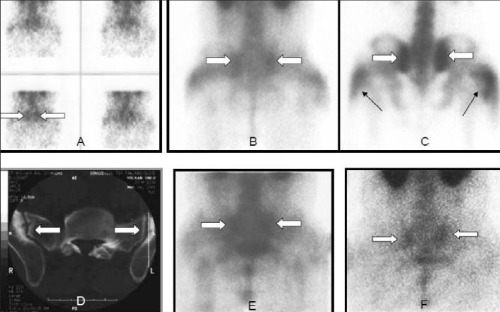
A 32-y-old male with clinically active sacroiliitis. Flow (A),blood pool (B), 4^th^ hour planar (C) images of bone scan. CT (D), 4thhour (E) and 24^th^ hour (F) HIG images of pelvis. Note the increasedflow, hyperemia and osteoblastic activity in sacroiliac joints on bonescintigraphy (white arrows). Increased HIG accumulation andretention were concordant with the clinical findings in this patient.Degenerative changes of both sacroiliac joints accompanied withsubchondral sclerosis and small calcifications were reported on CT(white arrows). The bilateral gluteal uptake is due to the repeatedintramuscular injections (black arrows)
